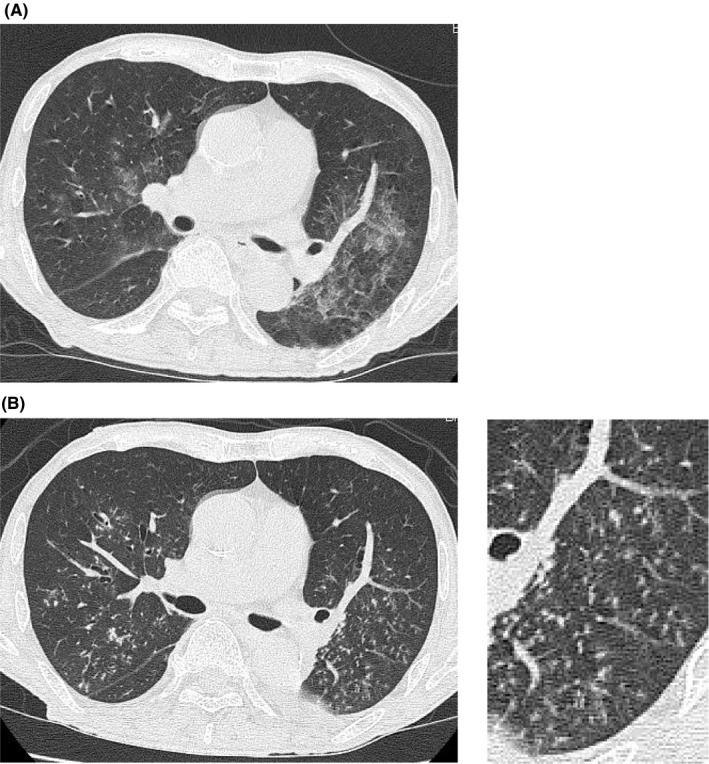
Computed tomographic images of the lungs. A, Computed tomography at admission shows notable infiltrative shadows along the airway in both lung fields. B, Computed tomography in hospital on day 9 shows left pleural effusion and centrilobular micronodules, as well as “tree‐in‐bud” branching opacities throughout both lung fields
